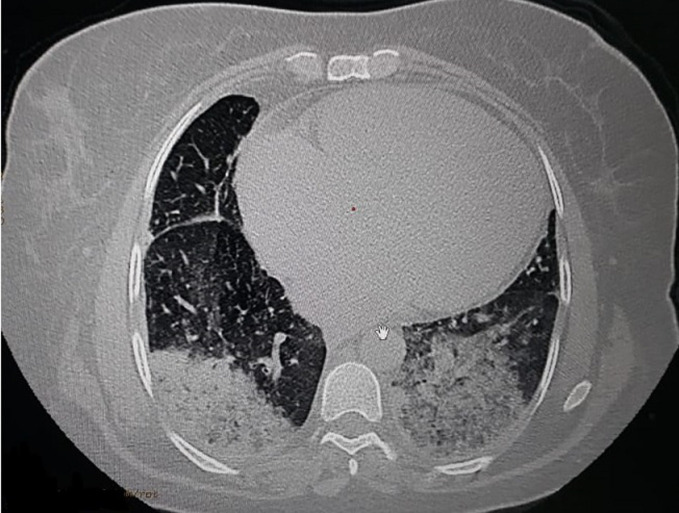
thoracic CT scan showing diffuse patchy ground-glass opacities suggesting COVID-19 pneumonia
